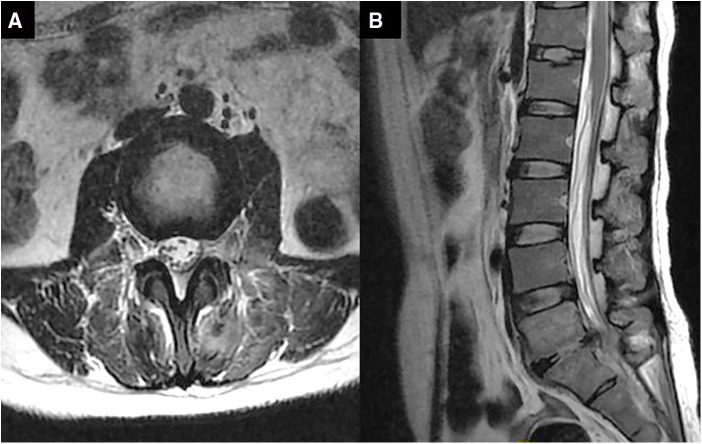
Magnetic resonance images of the lumbar vertebra after percutaneous endoscopic drainage for 1 month in axial (A) and sagittal (B). intraspinal and paravertebral abscesses have disappeared.
